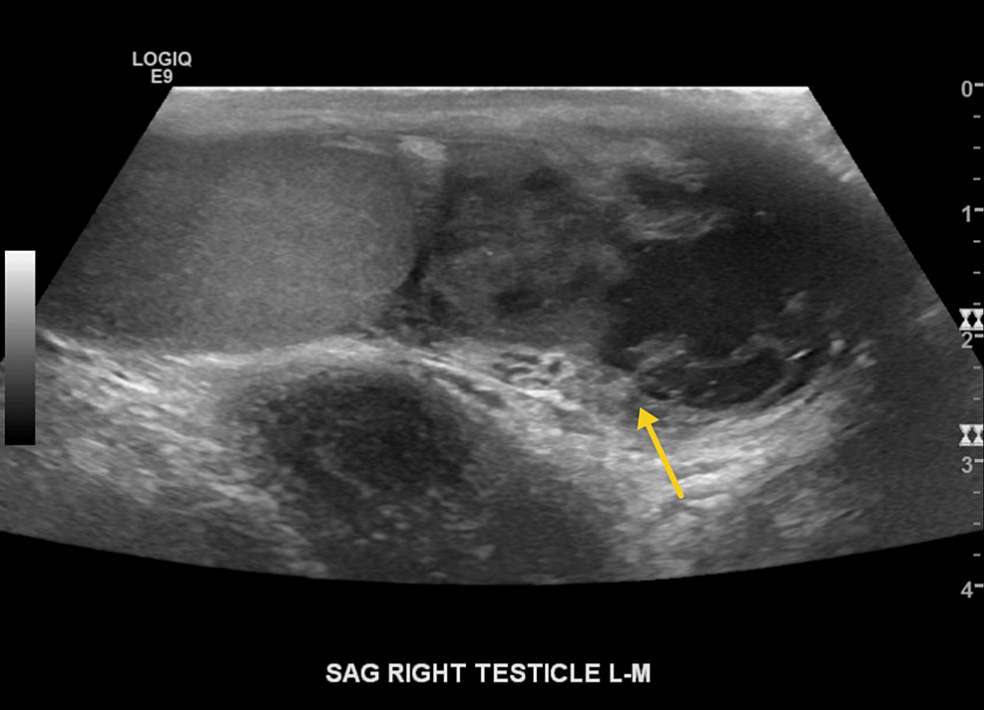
A representative grayscale sonographic image through the right testicle demonstrates development of new heterogeneous enlargement of the right epididymis with an extratesticular complex masslike area on the right testicle/epididymis.
